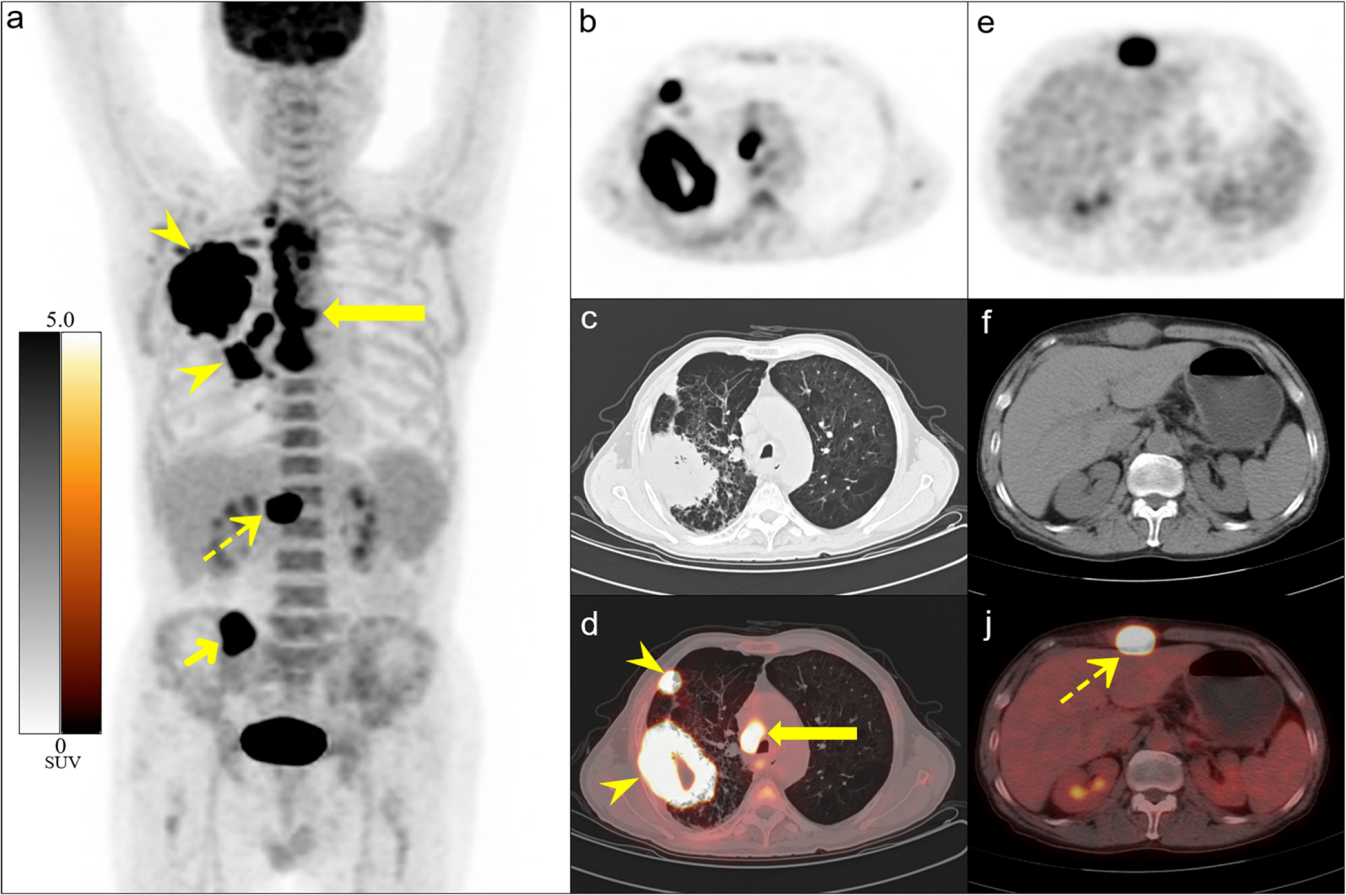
A case of lung adenocarcinoma with metastasis of right rectus abdominis as the first manifestation. A 64-year-old man presented with a 2-week history of a painful, tough mass in the upper abdomen, which was confirmed as metastatic adenocarcinoma by biopsy. ^18^F-FDG PET/CT imaging was performed to locate the primary tumor. Maximum intensity projection (MIP, a), chest axial images (b-d), and abdomen axial images (e-g) of PET/CT showed lesions in the upper lobe of the right lung (arrowheads), right rectus abdominis muscle (dotted arrows), multiple lymph nodes (long arrows) and right ilium (short arrow). Lung biopsy confirmed adenocarcinoma of the right lung. Therefore, a diagnosis of right lung cancer with lymph node, bone, and right rectus abdominis metastases was made. The patient survived for 6 months on palliative chemotherapy
